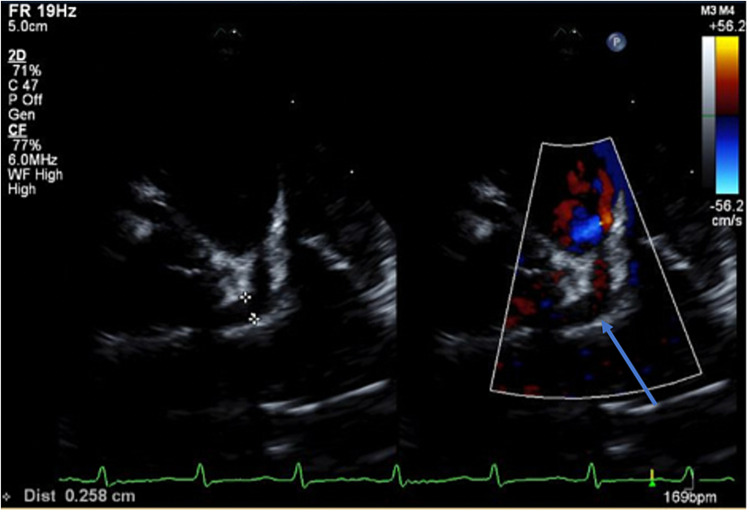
Transthoracic echocardiogram obtained on day 10 of illness showing a significantly dilated left main coronary artery (LMCA) (Z score = 3.4) (blue arrow)
